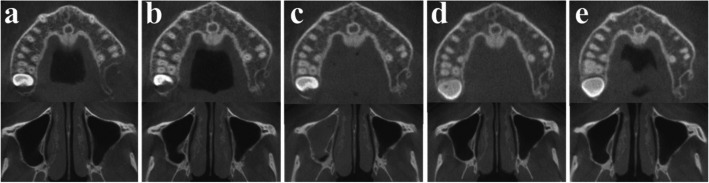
Regular follow-up of OKC after surgery using CBCT, (a) 3 months after surgery, (b) 1 year after surgery, (c) 2 years after surgery, (d) 3 years after surgery, (e) 5 years after surgery
